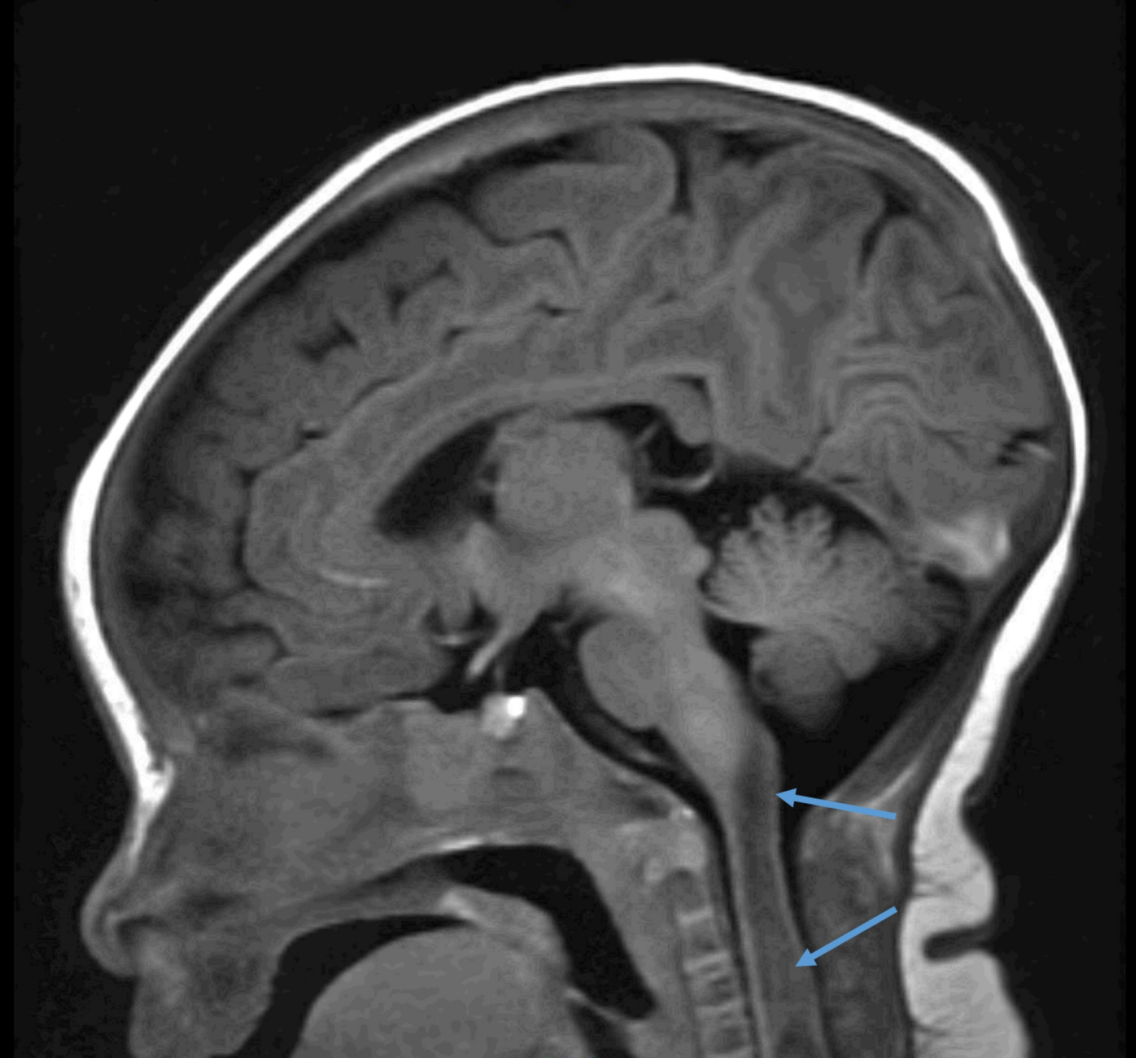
MRI Brain. MRI of the brain demonstrating abnormal signal enhancement in the proximal cervical spinal cord. MRI: Magnetic resonance imaging
